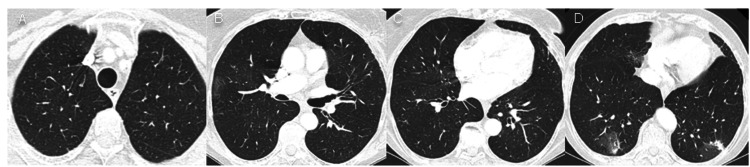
Chest CT 18 months after hospital discharge. Relapsing pulmonary consolidations and ground-glass-opacification areas in the lower lobes after steroid tapering.
